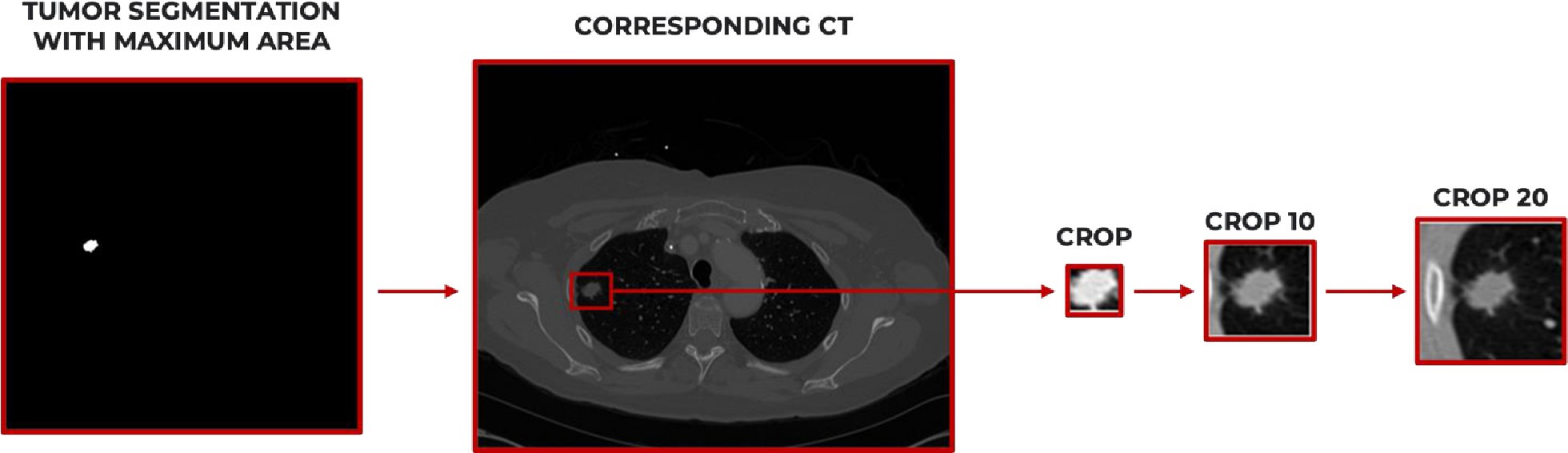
ROI extraction process. After identifying the tumor segmentation with maximum area, along with the corresponding CT image, three ROIs were extracted for each patient: CROP, CROP 10 and CROP 20.
